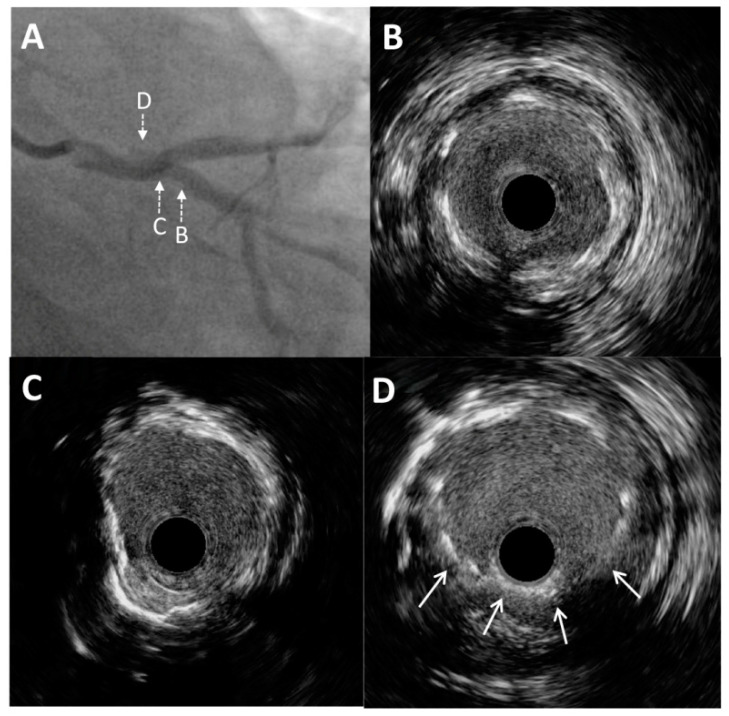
Intravascular ultrasound imaging of the left main and circumflex coronary artery. (A) Enlarged angiographic view of excessive stent protrusion from the circumflex artery to the left main. Pre-intervention cross-sectional intravascular ultrasound images from the proximal (B) and ostial (C) parts of the circumflex artery without significant restenosis and mild neointimal hyperplasia of a previously implanted stent. (D) Visualization of “floating struts”—excessive stent protrusion from the circumflex coronary artery into the left main (arrows).
